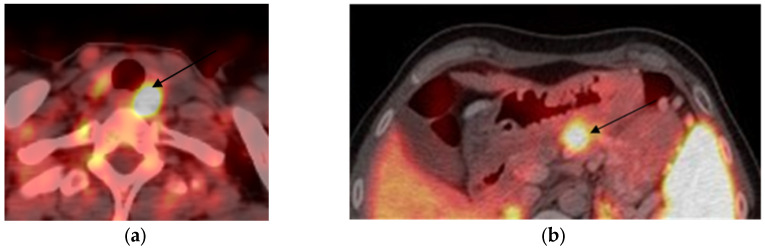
[68Ga]-DOTA-TATE PET/CT-scans: (a) [68Ga]-DOTA-TATE positive 11 × 7 × 13 mm lesion corresponded to left upper parathyroid adenoma (arrow); (b) [68Ga]-DOTA-TATE positive 37 × 30 × 36 mm lesion in pancreas head (arrow).
